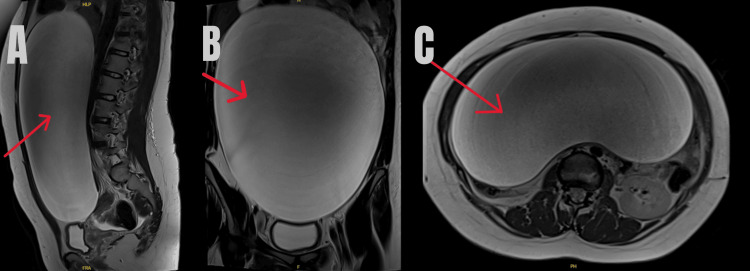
MRI of the abdomen and pelvis demonstrating a giant cystic lesion. The red arrow indicates the large cyst. (A) Sagittal T2-weighted MRI image showing a large, well-defined, homogeneously hyperintense cystic lesion occupying the abdominopelvic cavity, extending from the pelvis to the upper abdomen and causing posterior displacement of bowel loops and adjacent structures. (B) Coronal T2-weighted MRI image demonstrating the full craniocaudal extent of the cystic mass, with a smooth thin wall and no internal septations or solid components, exerting mass effect on surrounding abdominal organs. (C) Axial (transverse) T2-weighted MRI image showing a large, unilocular, hyperintense cyst with a thin wall, compressing adjacent bowel loops and retroperitoneal structures without evidence of invasion.
